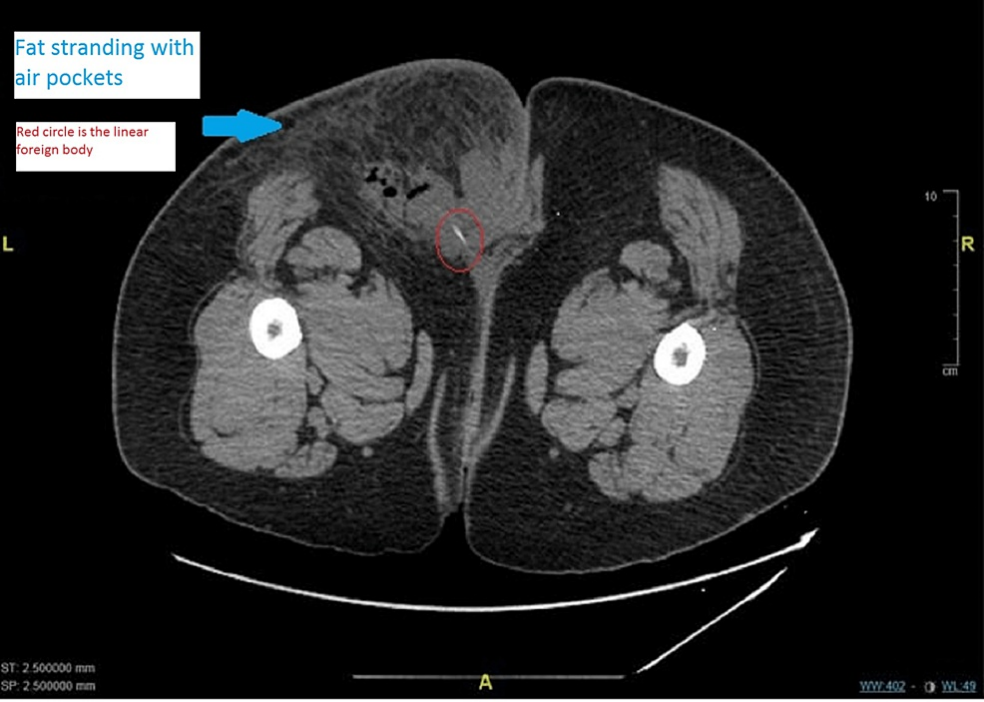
CT scan of pelvis (prone position, axial section) showing linear foreign body (red circle) in left gluteal region abscess with air pockets and subcutaneous fat stranding (blue arrow)
